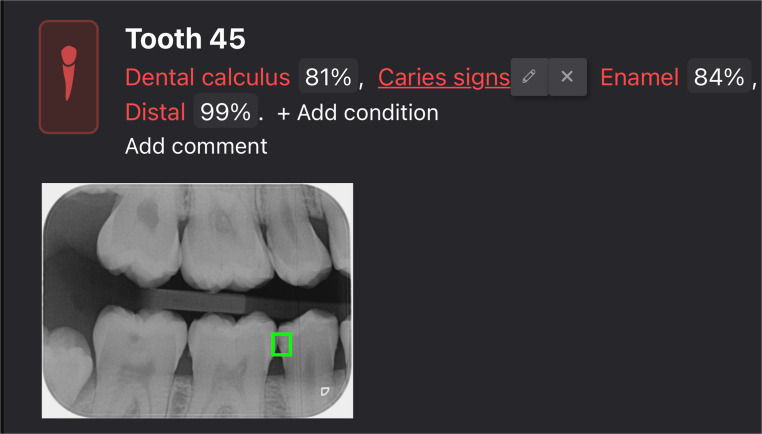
Example of Diagnocat™ classification of detected caries on a bitewing radiograph. Lesions are categorized by the software into “enamel”, “dentin”, or “secondary caries,” with visual color-coded overlays and accompanied by probability scores. This classification is clinically relevant, as differentiation between the depth of enamel and dentin lesions informs treatment planning and risk assessment
